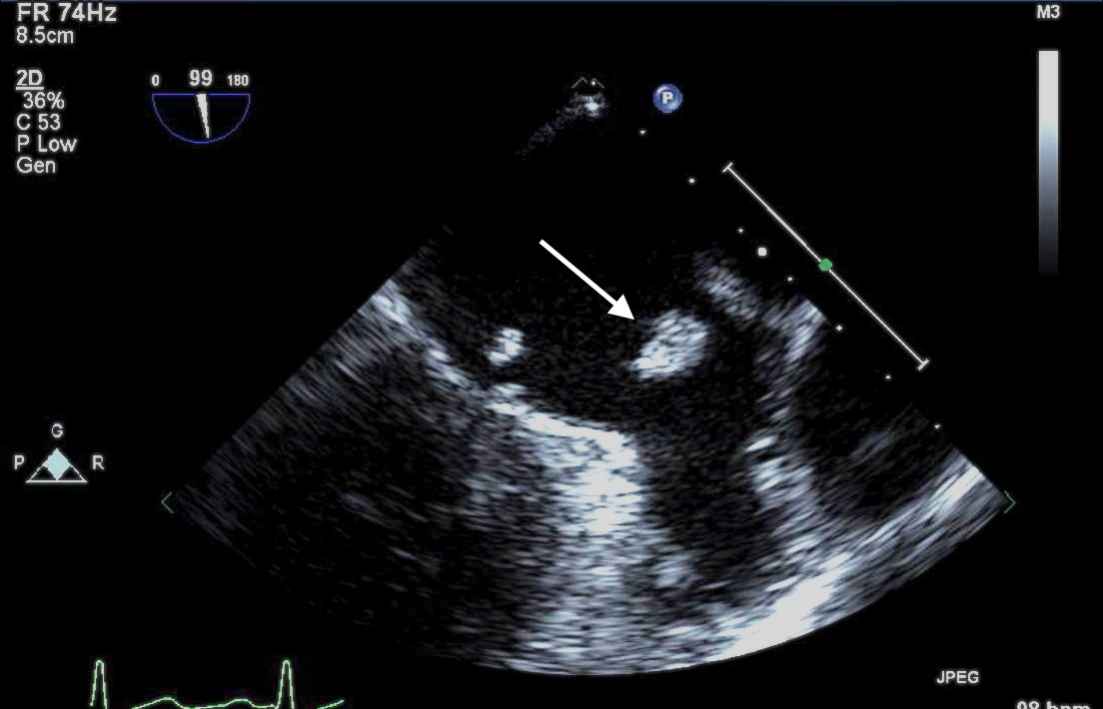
Transesophageal echocardiogram (TEE) showing the mitral valve vegetation (arrow)
